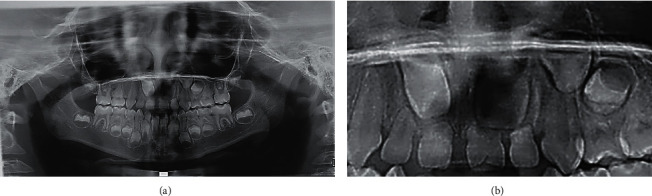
Panoramic X-ray shows teeth 17-11-21-23-24 and 27 in intraosseous formation, in Nolla stage between 3 and 5. The formation of the other maxillary permanent teeth is not appreciated, indicating their agenesis (a and b). Panel (b) shows a close-up view of the anterosuperior area. The formation of teeth 12, 22, and 13 is not appreciated.
